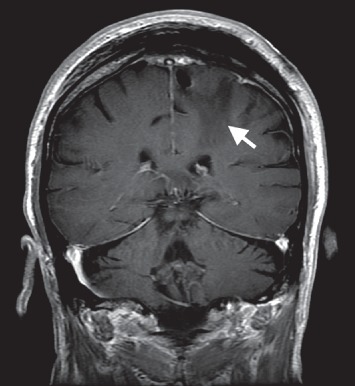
Postresection, T1 postgadolinium MRI brain with coronal view. This reveals postsurgical changes after a gross total resection of the left frontoparietal mass with residual edema in the tumor bed.
